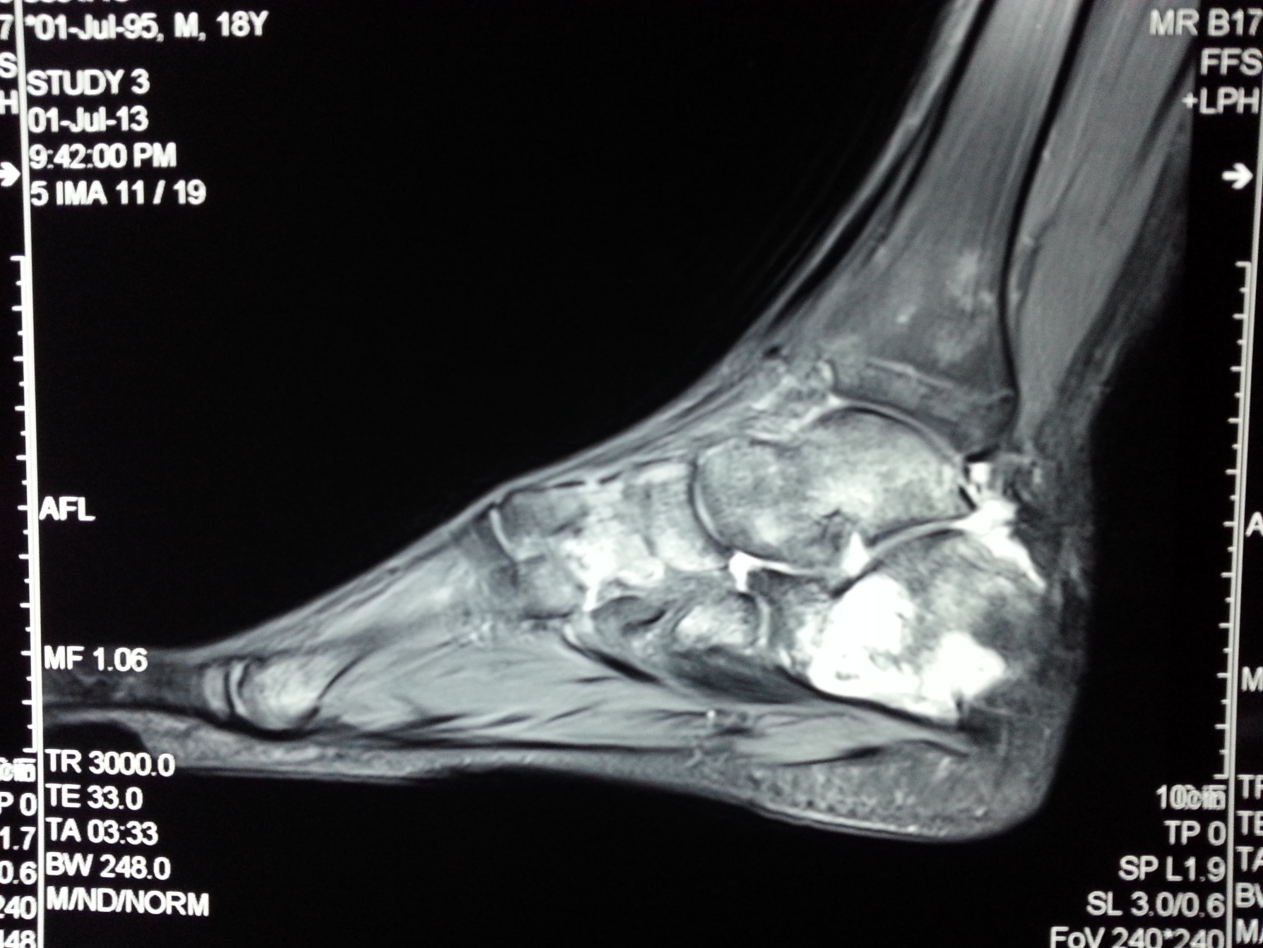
MRI of the same case showing fluid in subtalar joint and signal changes in most hindfoot bones.
